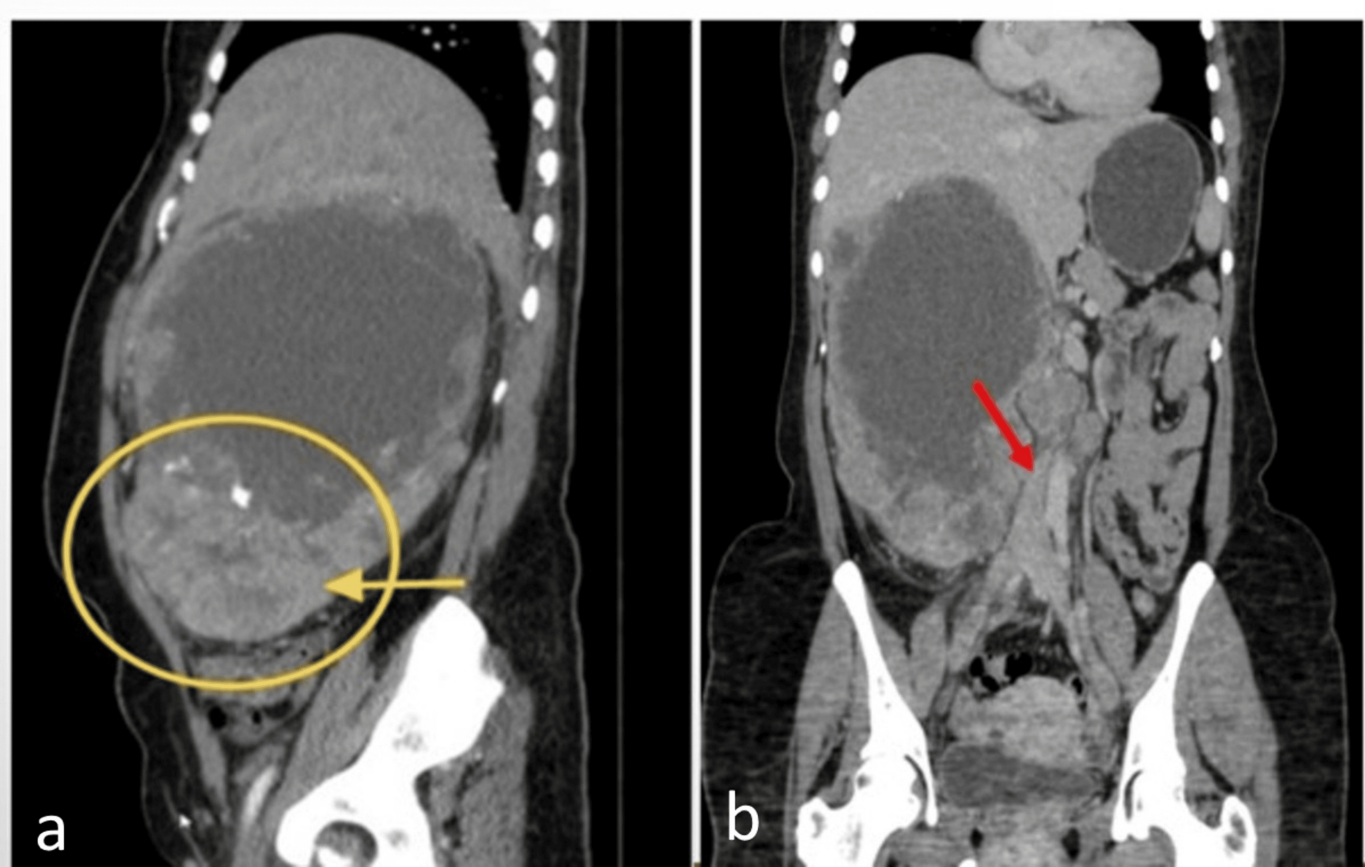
Sagittal CT arterial phase (a) and coronal CT venous phase (b) shows a large heterogeneously enhancing lesion with areas of necrosis. Suspicious reniform structure in the lower pole (yellow circle). Non-visualization of the upper half of the IVC, with the distal half of the IVC seen (red arrow). CT: computed tomography, IVC: inferior vena cava
